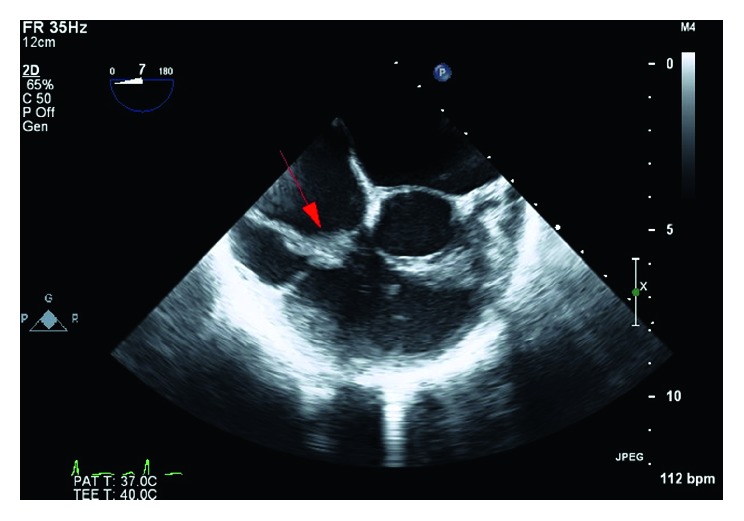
Postprocedural transesophageal echocardiogram (TEE). Midesophageal short-axis 0-degree view revealing the tricuspid valve vegetation reduced in size to 2.1 cm from its largest diameter (red arrow).
